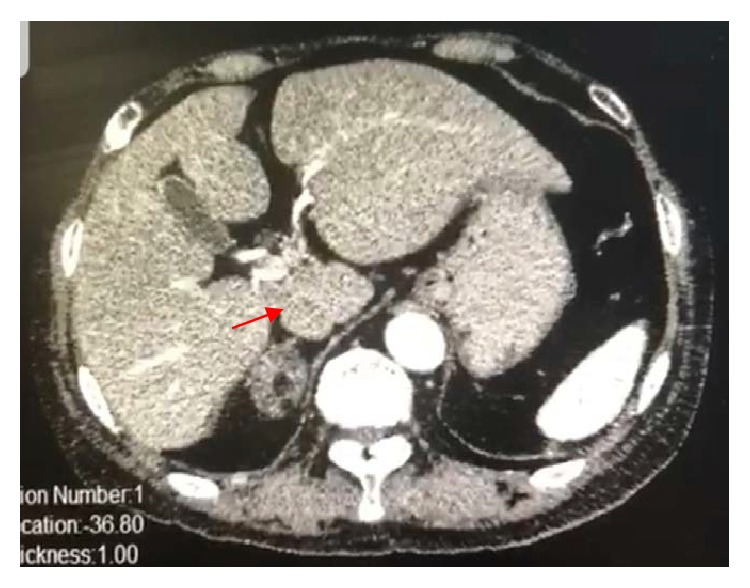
CT scan adrenal showing right adrenal adenoma.
